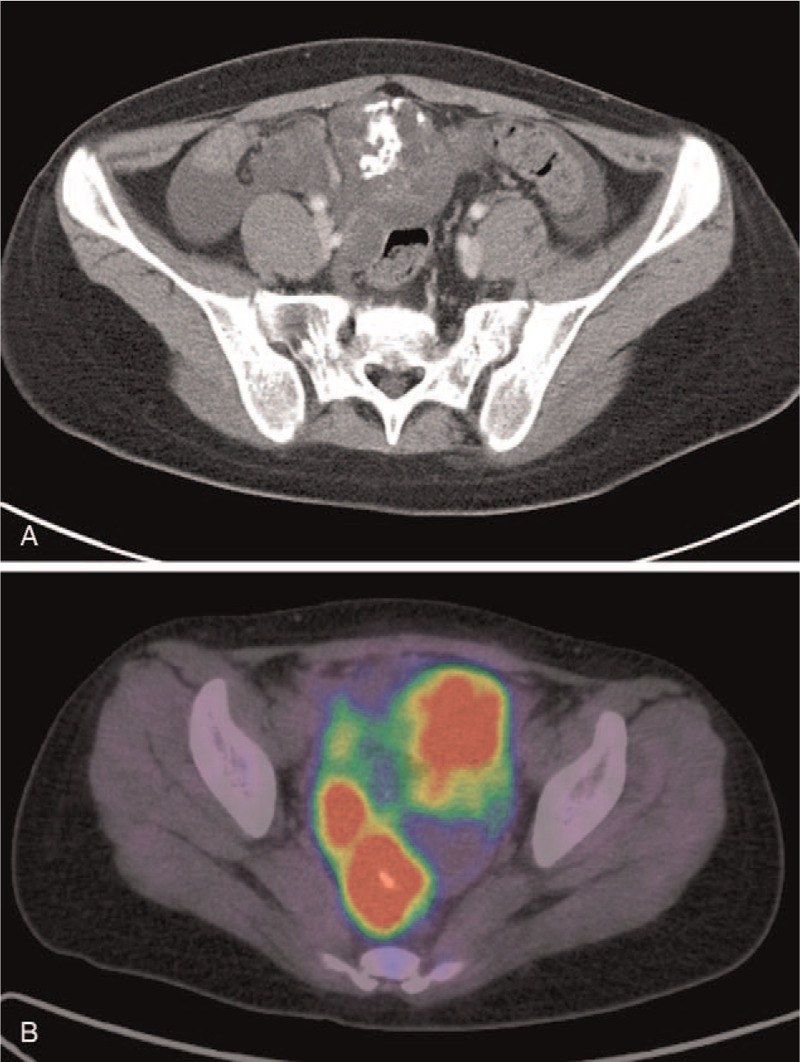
Post-chemotherapy findings from abdominal computed tomography (A) and positron emission tomography–computed tomography (B). The tumor had grown (A), and abnormal accumulation was observed (B).
